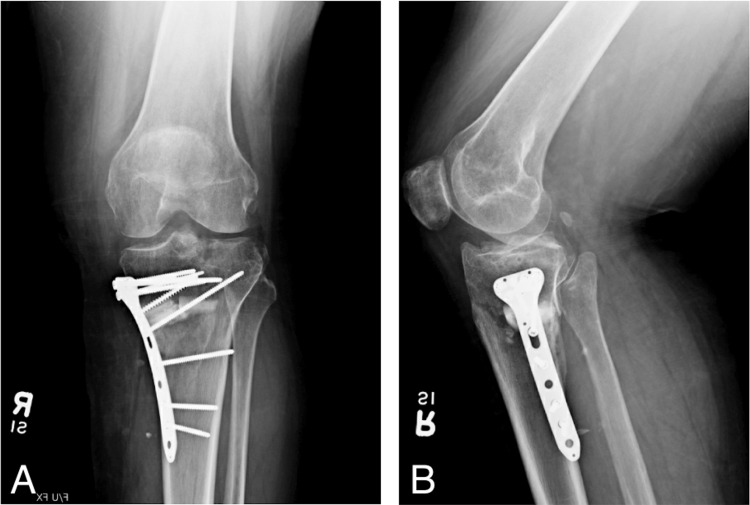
One-month follow-up X-rays of the right knee demonstrating intact hardware with appropriate alignment of the knee and fracture fragments.
